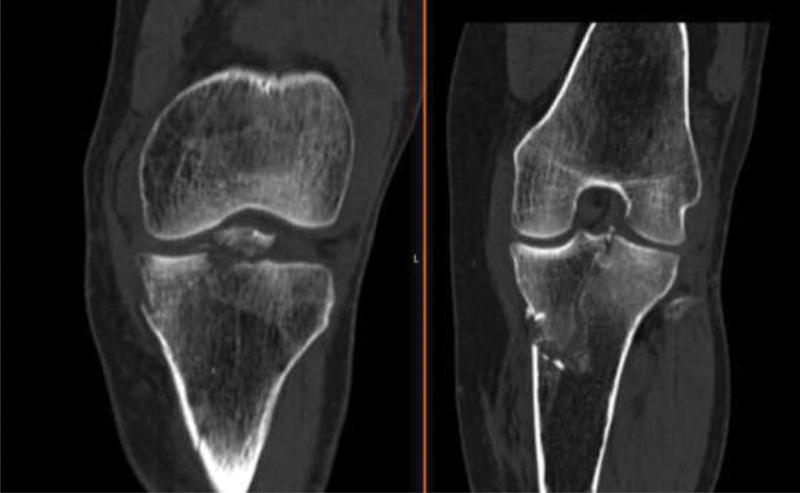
Coronal cuts of the knee computed tomography scan.
